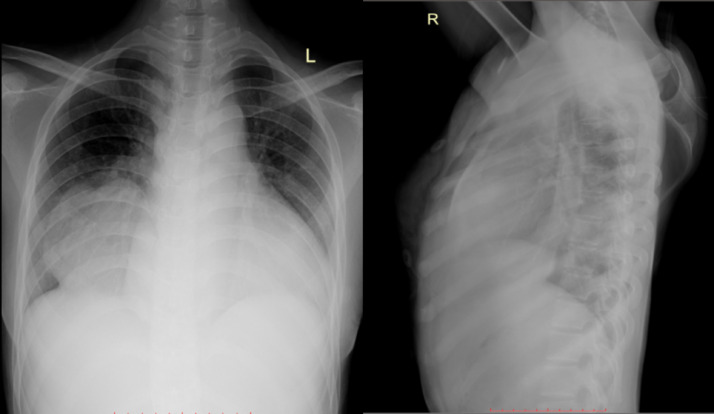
PA chest X-ray shows a mass obscuring the right hemidiaphragm, while the lateral view demonstrates a mass in retrocardiac area.
